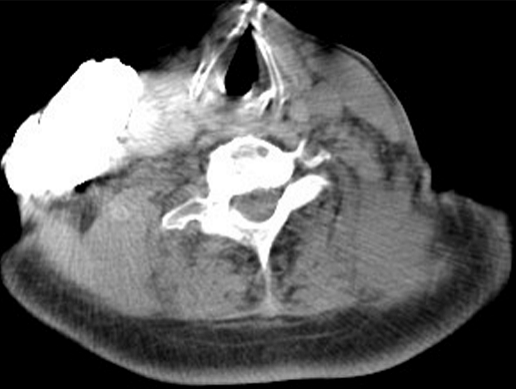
Axial contrast-enhanced chest CT scan demonstrates extravasation of non- ionic CM into the right subcutaneous cervical region
